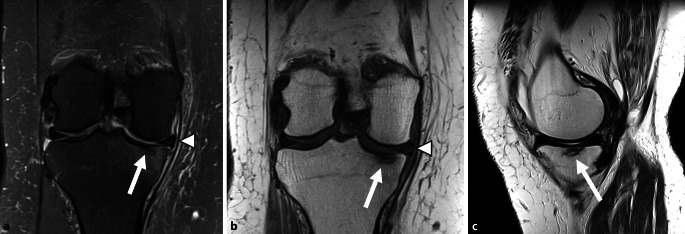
A reversible, low grade subchondral insufficiency fracture of the knee in the medial tibial plateau. a A coronal fluid sensitive magnetic resonance image. b A coronal T1WI. c A sagittal Proton Density weighted imaging. The classical triad of diffuse bone marrow edema, a subchondral fracture line (*arrow*) and medial meniscus body extrusion (*arrowhead*) is noted
